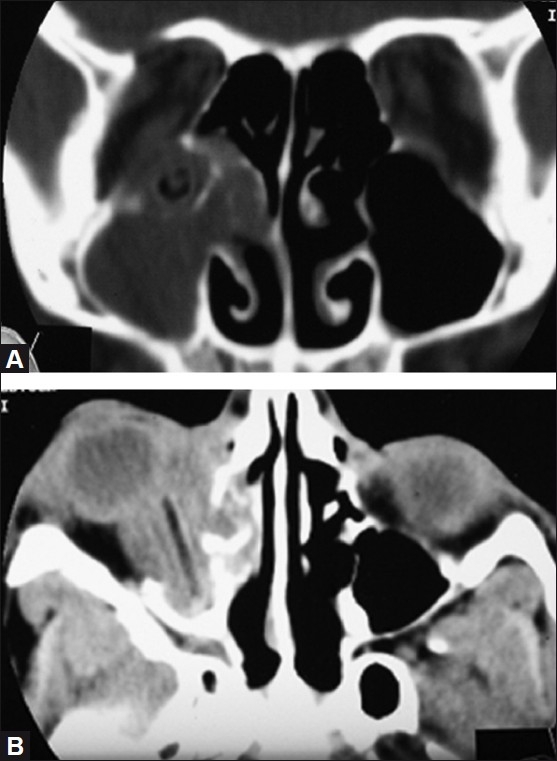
(A) Coronal non-contrast computed tomography (CT) scan showing the foreign body piercing the floor of the orbit into the maxillary sinus. (B) Axial non-contrast CT scan showing the entire extent of the foreign body
